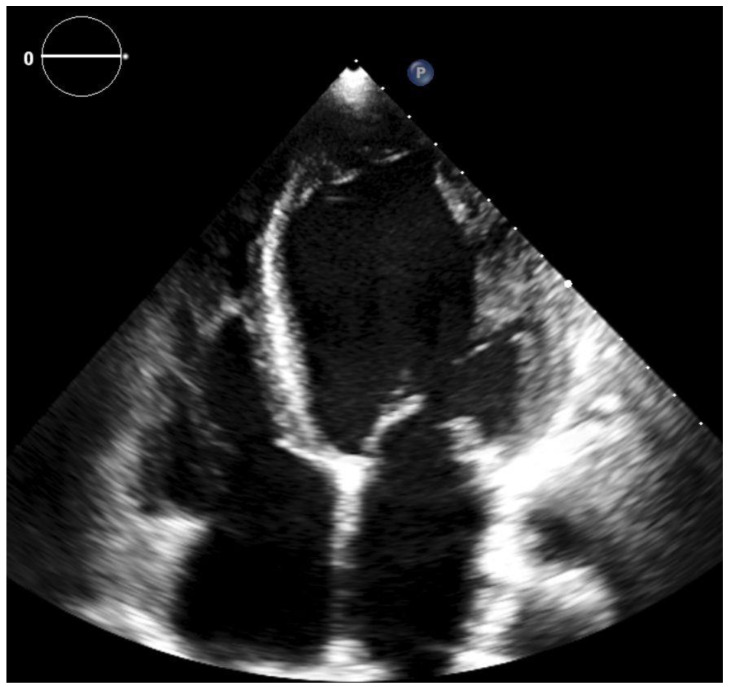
2D transthoracic echocardiography, apical four chamber view (second examination)—no thrombi are visible in the left ventricular cavity.
